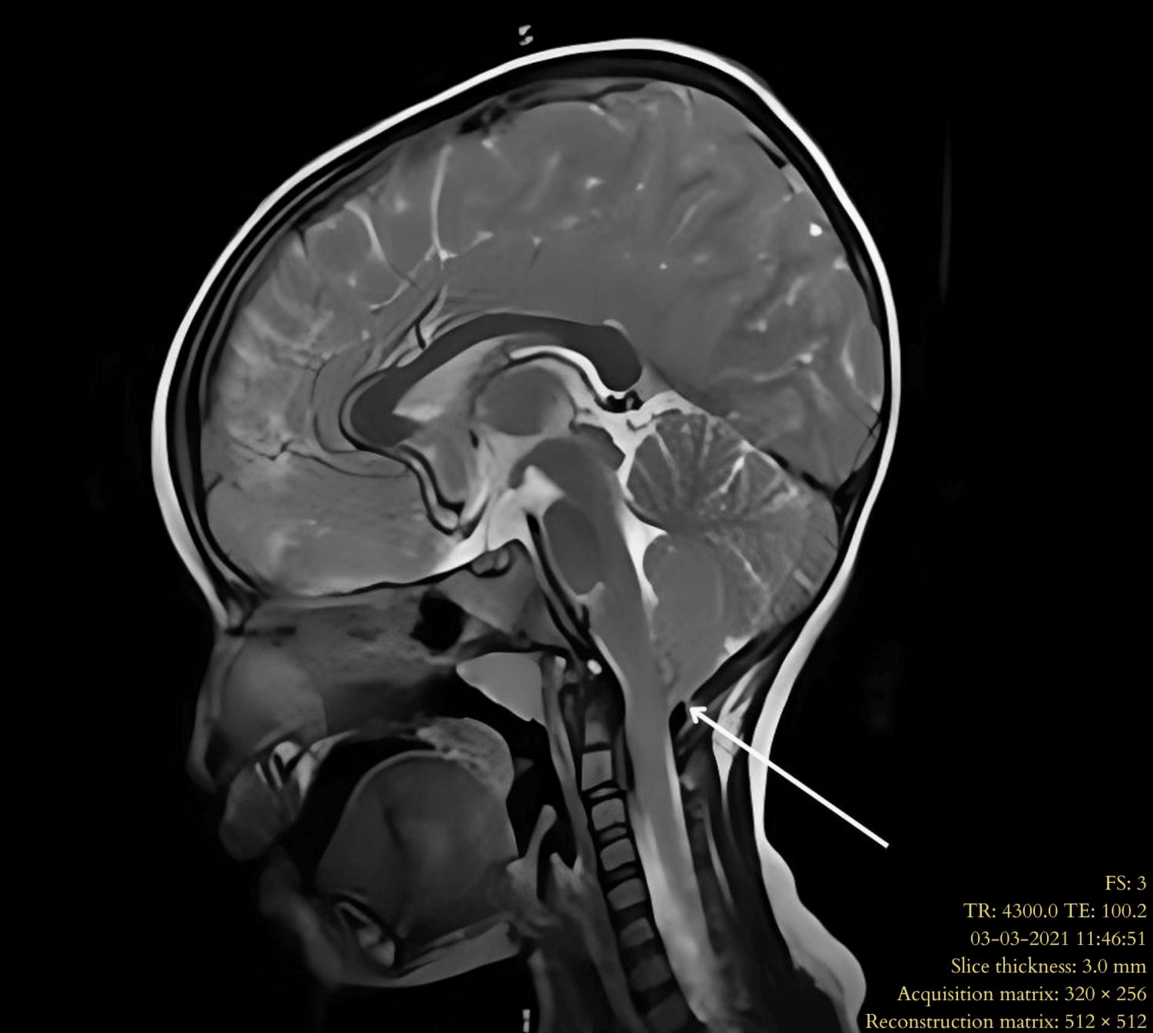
MRI sagittal T2-weighted sequence of the brain in Type 1 Chiari malformation showing a peg-shaped cerebellar tonsil (white arrow) with a crowded foramen magnum.
